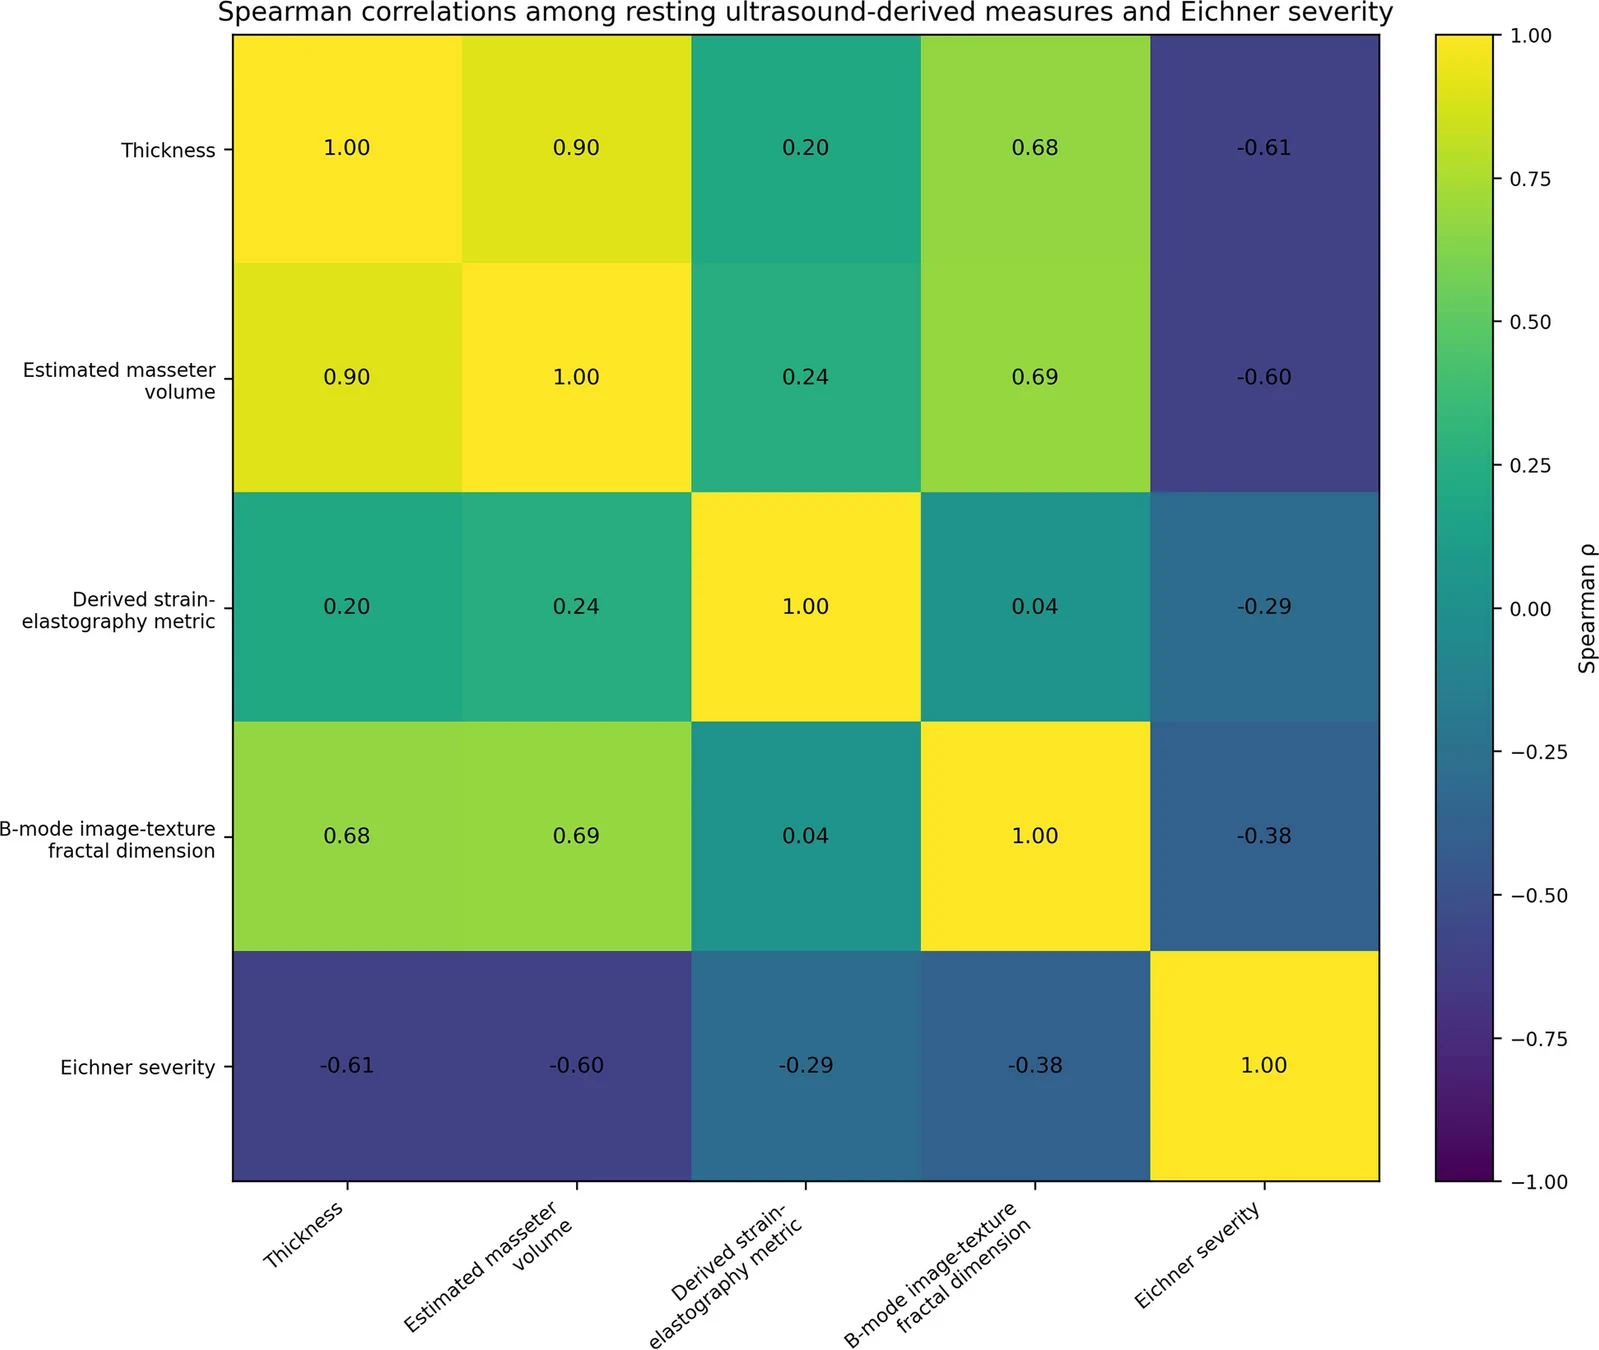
Spearman correlation heatmap of resting ultrasound-derived measures and Eichner severity. Values are Spearman coefficients calculated from participant-level resting bilateral means. All correlation analyses were exploratory, and findings involving B-mode image-texture fractal dimension should be considered hypothesis-generating
